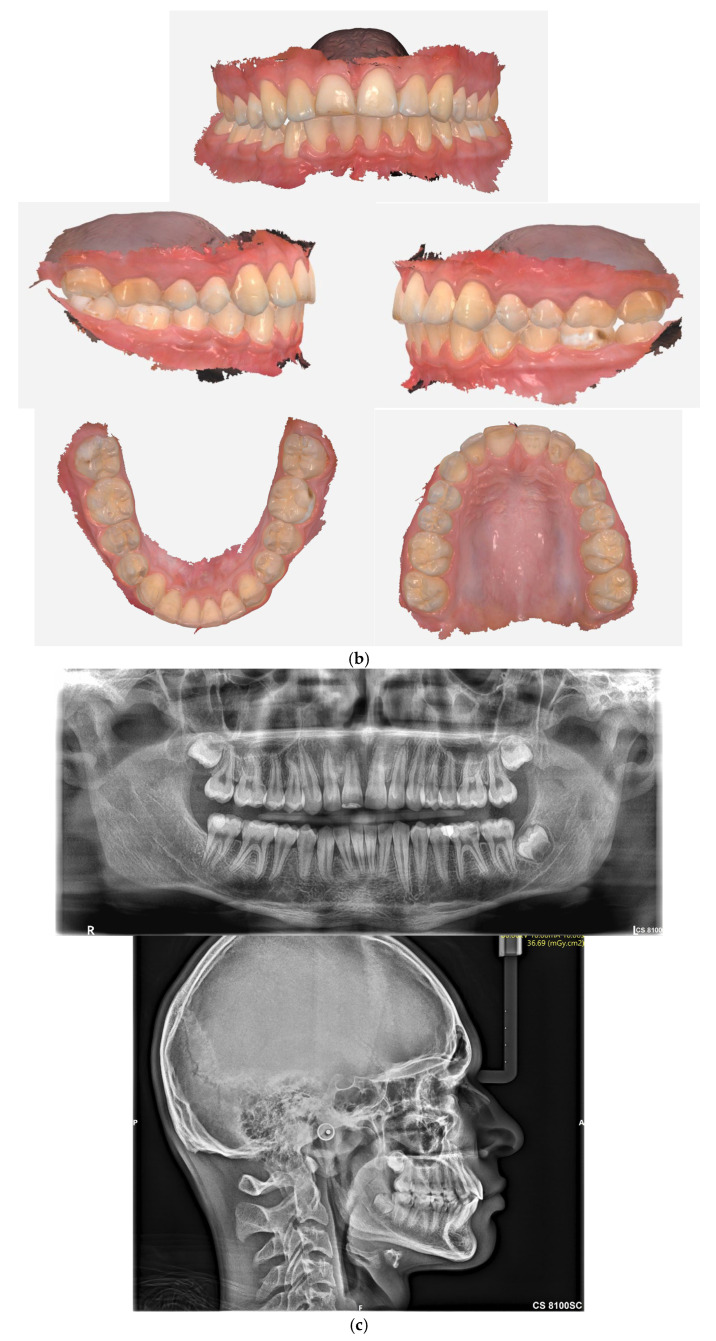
Intraoral photos (a), models (b), and X-rays (c) after treatment.
